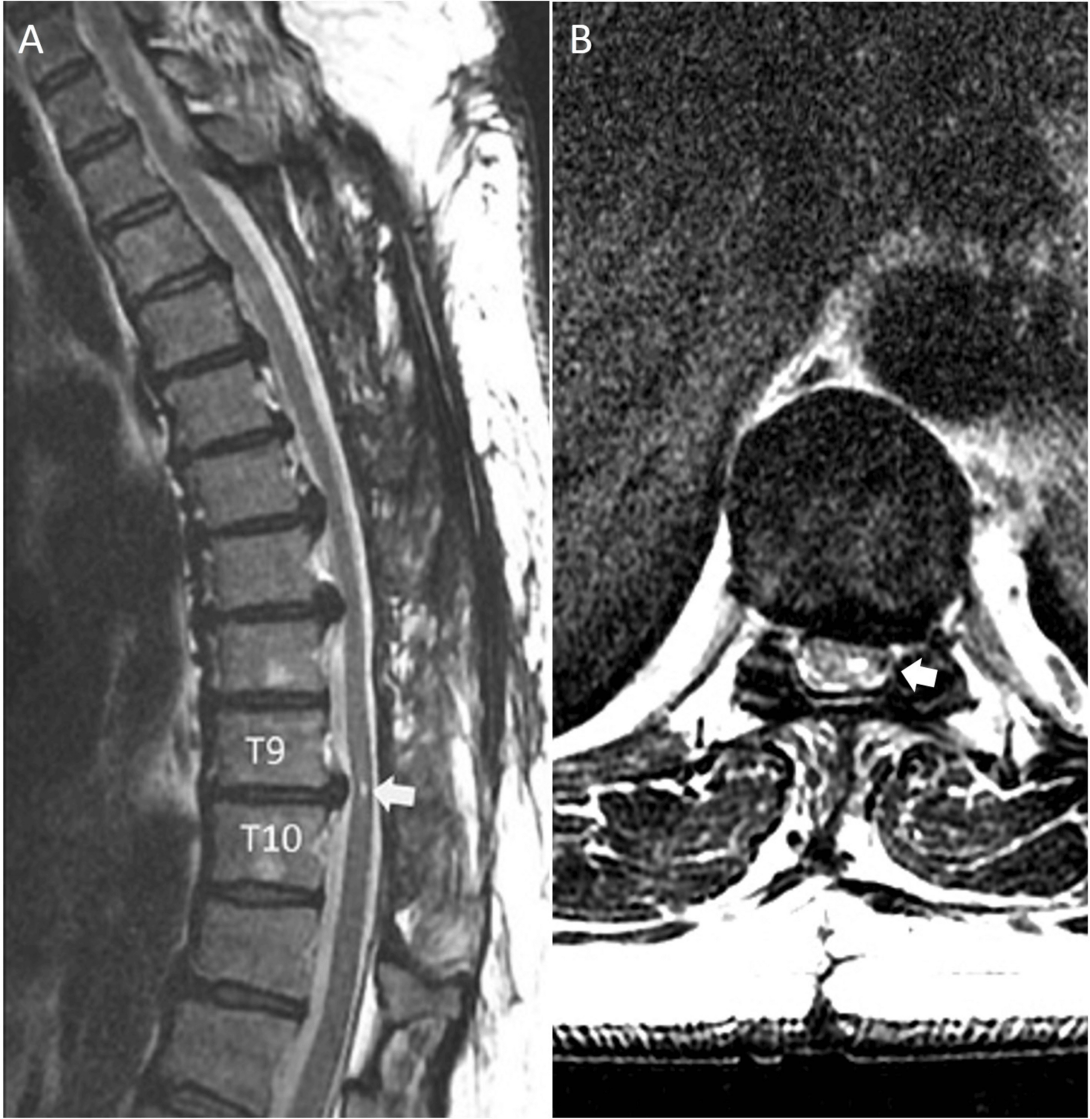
Postoperative two-year MRI of the thoracic spine revealed well decompression of the thoracic spine (A) Sagittal view. Myelomalacia (arrow) was noted at T9/10 level. (B) Axial view. T9/10 level. Myelomalacia (arrow) was noted.
